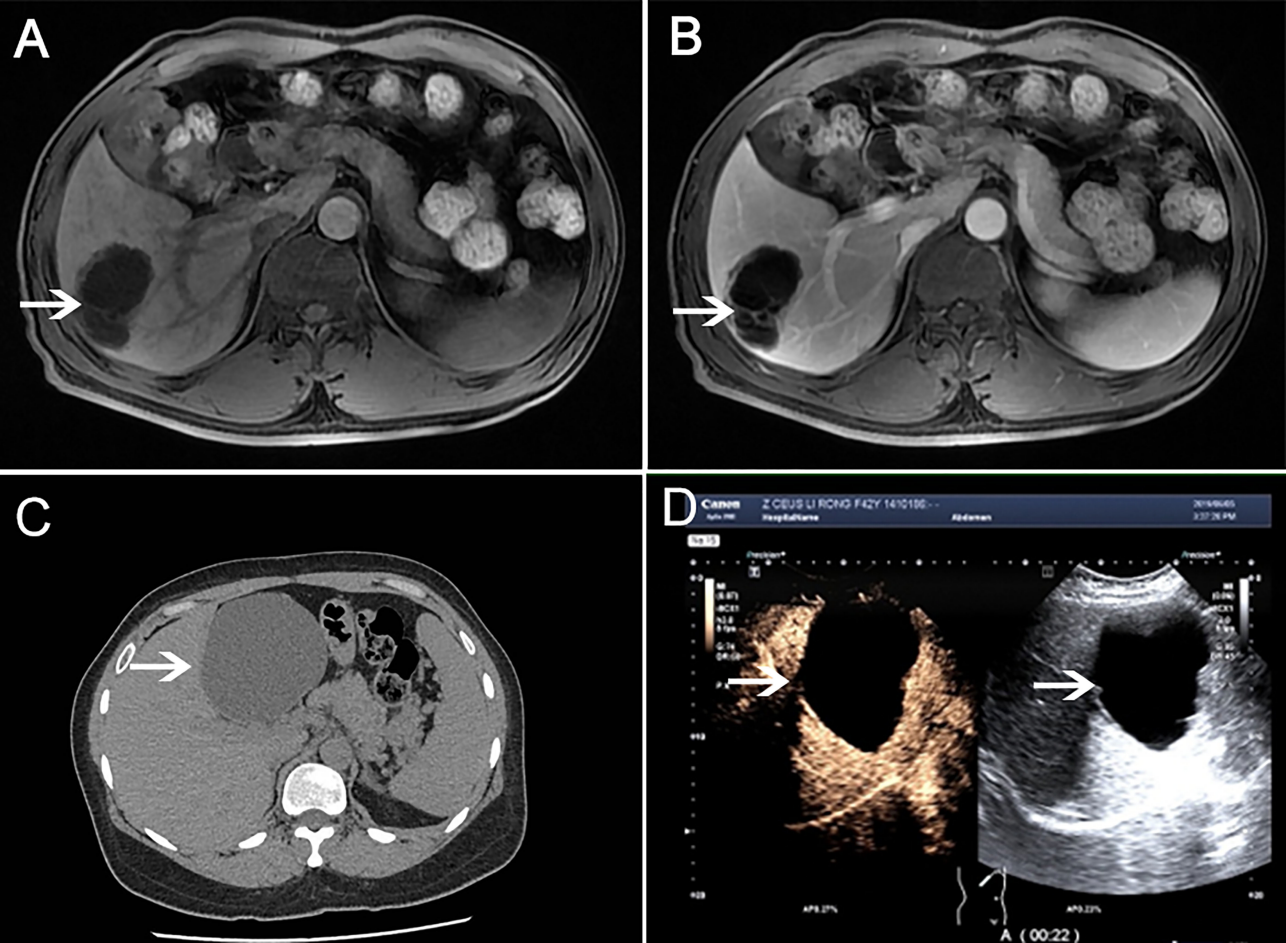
MRI examination (Case 2). (A) Multilocular cystic mass in the liver’s right lobe (shown by white arrow). (B) Contrast-enhanced T1-weighted images showed that the cyst wall was separated and enhanced, and there was no nodule on the cyst wall, and the contents of the lesion were not enhanced in portal vein phase. CT examination (Case 6). (C) A round low-density shadow is seen in the left lobe of the liver, with clear edges and uniform density in the capsule. (D) Contrast-enhanced liver ultrasound showed cystic space occupying the left outer lobe of the liver, with clear borders, good sound penetration in the cyst, and floating sheet echoes in the local area. There was no enhancement in the cysts at various stages after injection of contrast agent.
